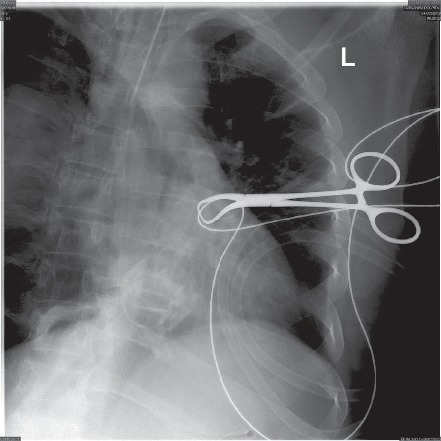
Intraoperative chest radiograph showing normal left lung field.
